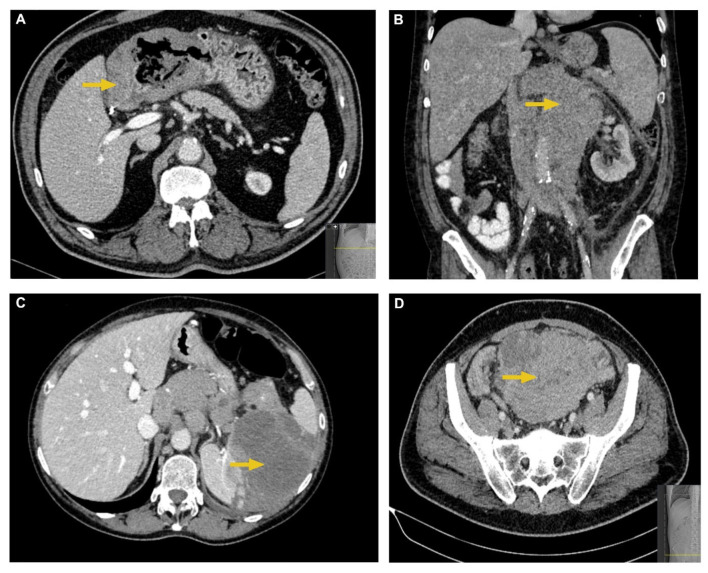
Typical MSCT characteristics of abdominal lymphomas. (A) Axial postcontrast CT image shows irregular, circumferential, and hypoattenuating thickening of antral and pyloric gastric wall in a patient with DLBCL. (B) Coronal postcontrast CT image shows an extensive, retroperitoneal lymph node conglomerate which encapsulates the aorta and inferior vena cava in a patient with FL. (C) Axial postcontrast CT image shows a large hypodense and hypoenhancing splenic mass in a patient with DLBCL. (D) Axial postcontrast CT image shows a large, heterogeneous intraperitoneal mass which displaces the bowel loops in a patient with DLBCL.
